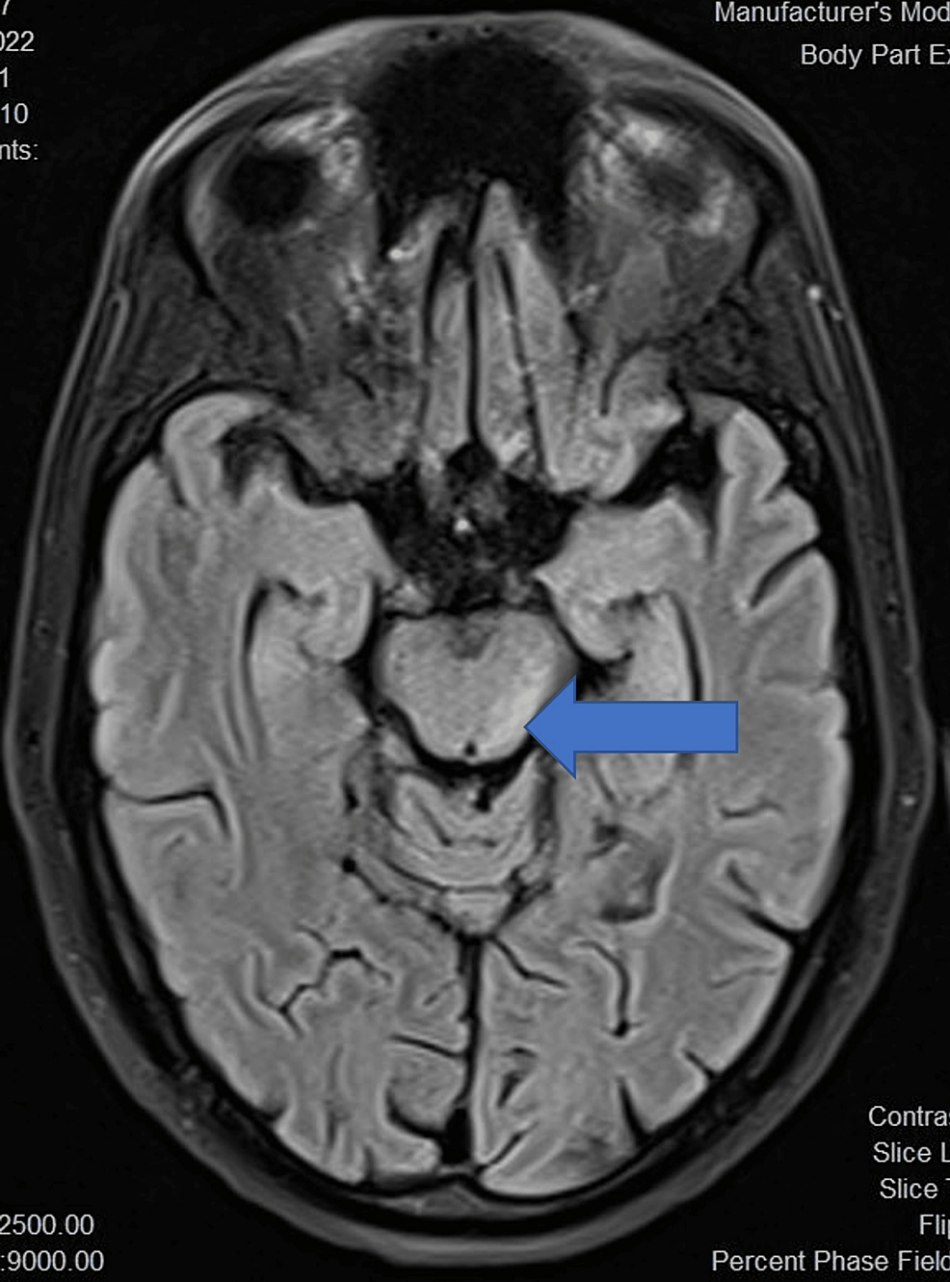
T2 FLAIR axial image shows patchy hyperintensity in midbrain FLAIR: fluid-attenuated inversion recovery
